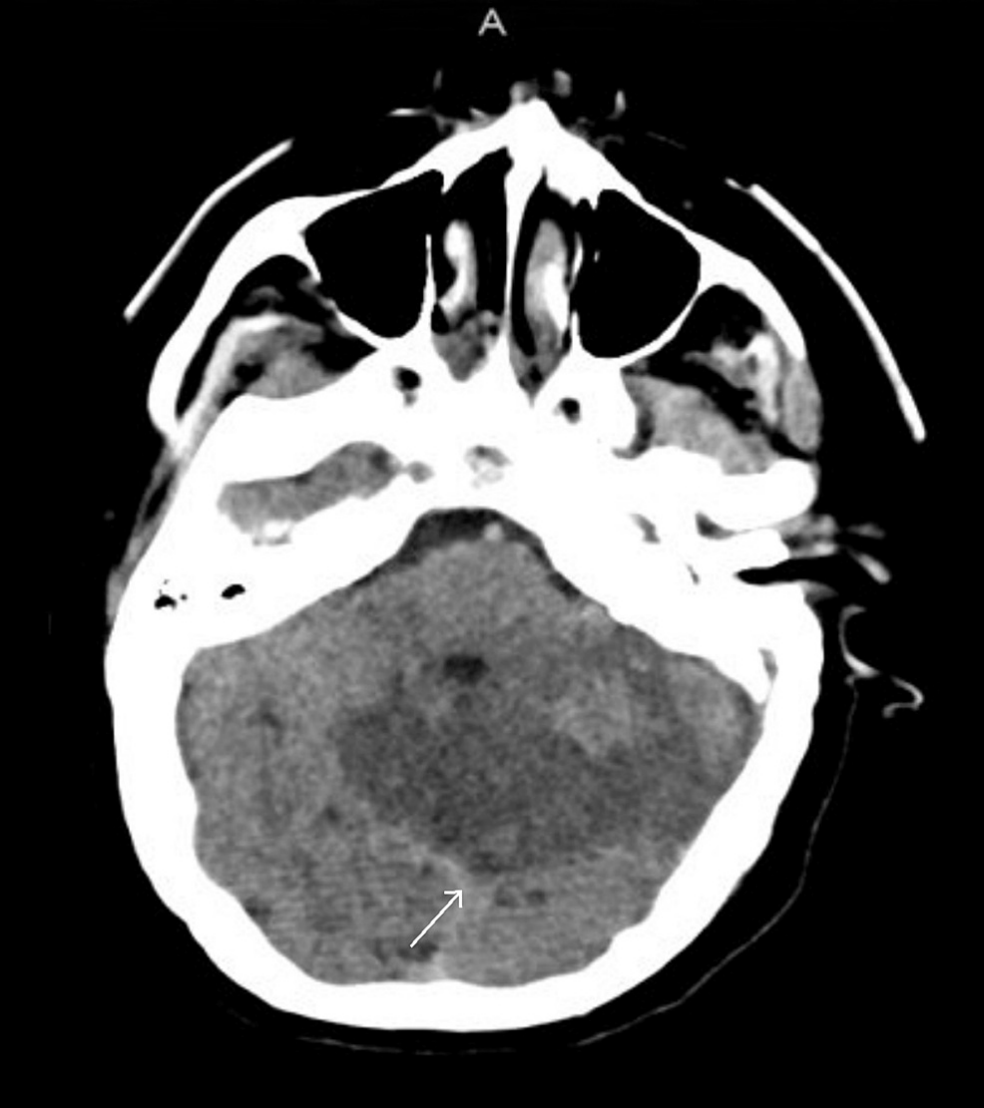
CT head showing left cerebellar infarct CT: Computed tomography
